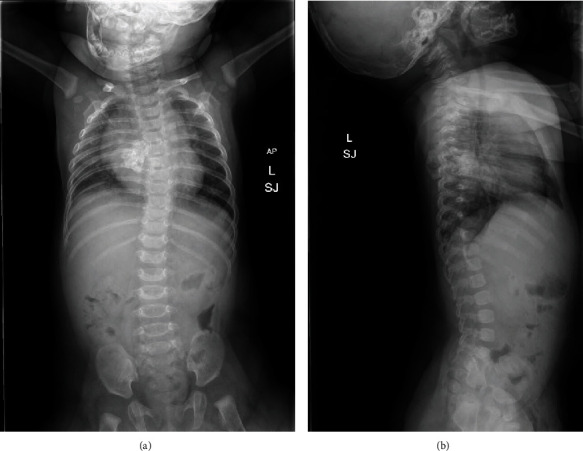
(a) AP spine radiograph showing thoracic levoscoliosis with a calcified lesion projecting from the right posterior aspect of the midthoracic rib segments extending into the soft tissues. (b) Sagittal spine radiograph further demonstrating osseous projection from the midthoracic posterior rib segments.
